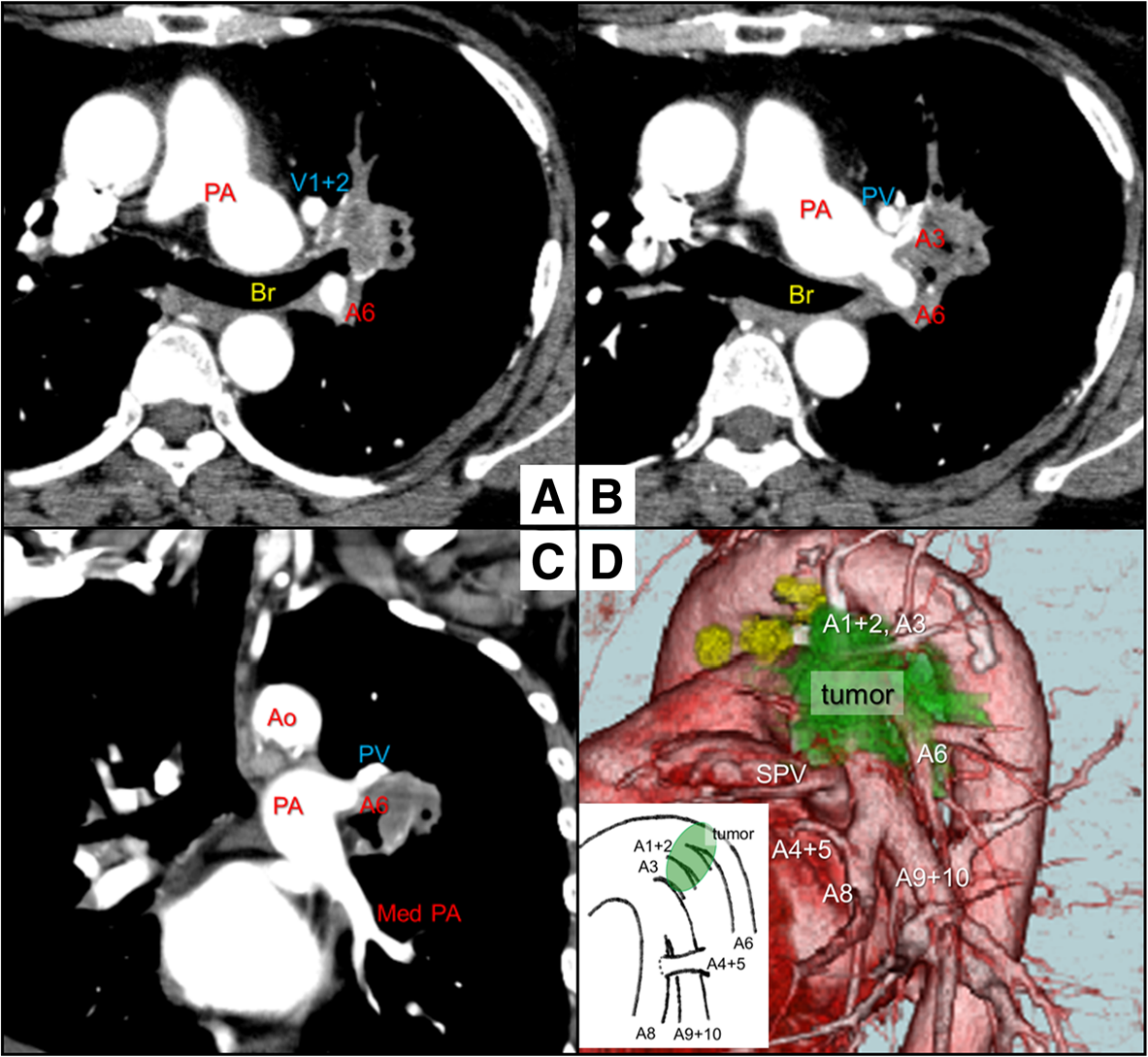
Chest computed tomography (CT) (a, b axial view and c coronal view) demonstrated the tumor invading the left upper bronchus in the left upper lung lobe, and the A3 and A6 branches were involved in the tumor. d Three-dimensional CT revealed that pulmonary artery branches (A4 + 5, A8, and A9 + 10) extended into the dorsal side of the superior pulmonary vein
